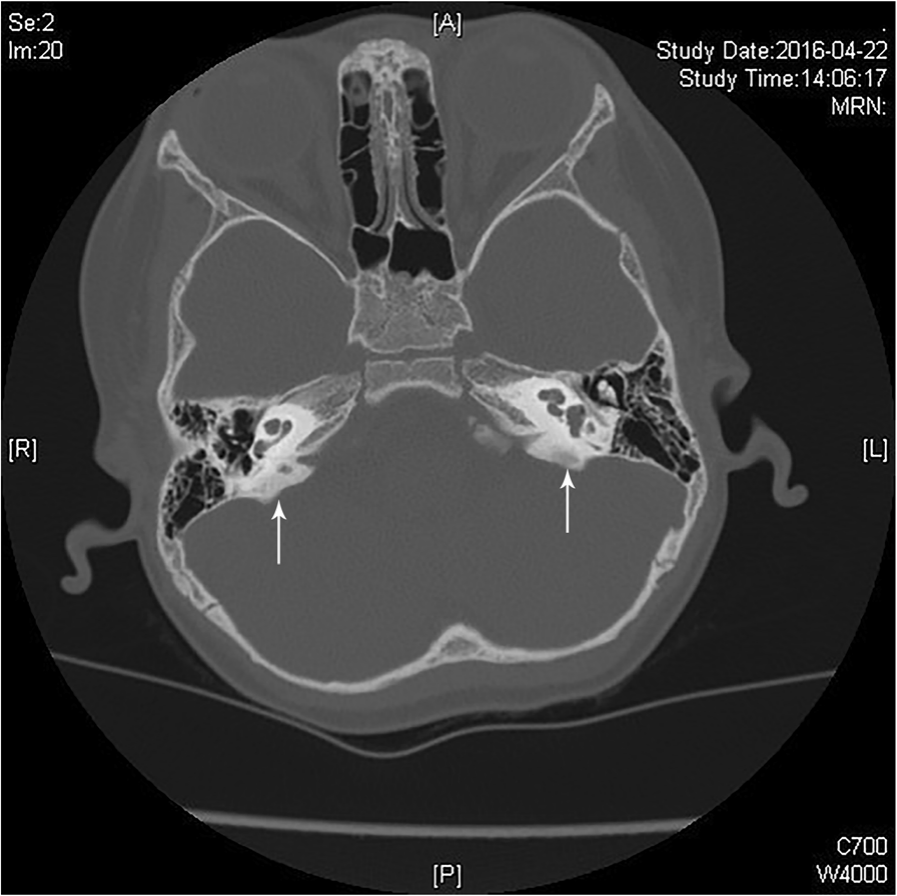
High-resolution computed tomography indicated bilateral enlargement of the vestibular aqueduct
